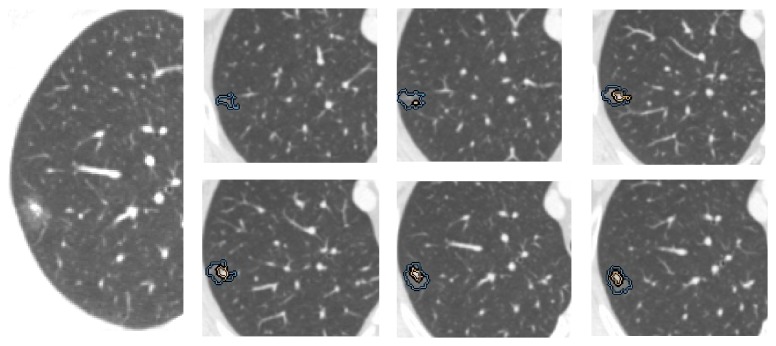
CT images use semiautomated segmentation and quantification of GGO volume percentage in a lepidic predominant invasive adenocarcinoma with EGFR mutation and GGO-containing nodule in a 65-year-old female. The tumor outer contour (blue line) and contour of the inner solid nodule (yellow line) are overlapped on each transverse image. The total tumor volume is 1.08 cm^3^ and the GGO volume percentage is 71.3%.
